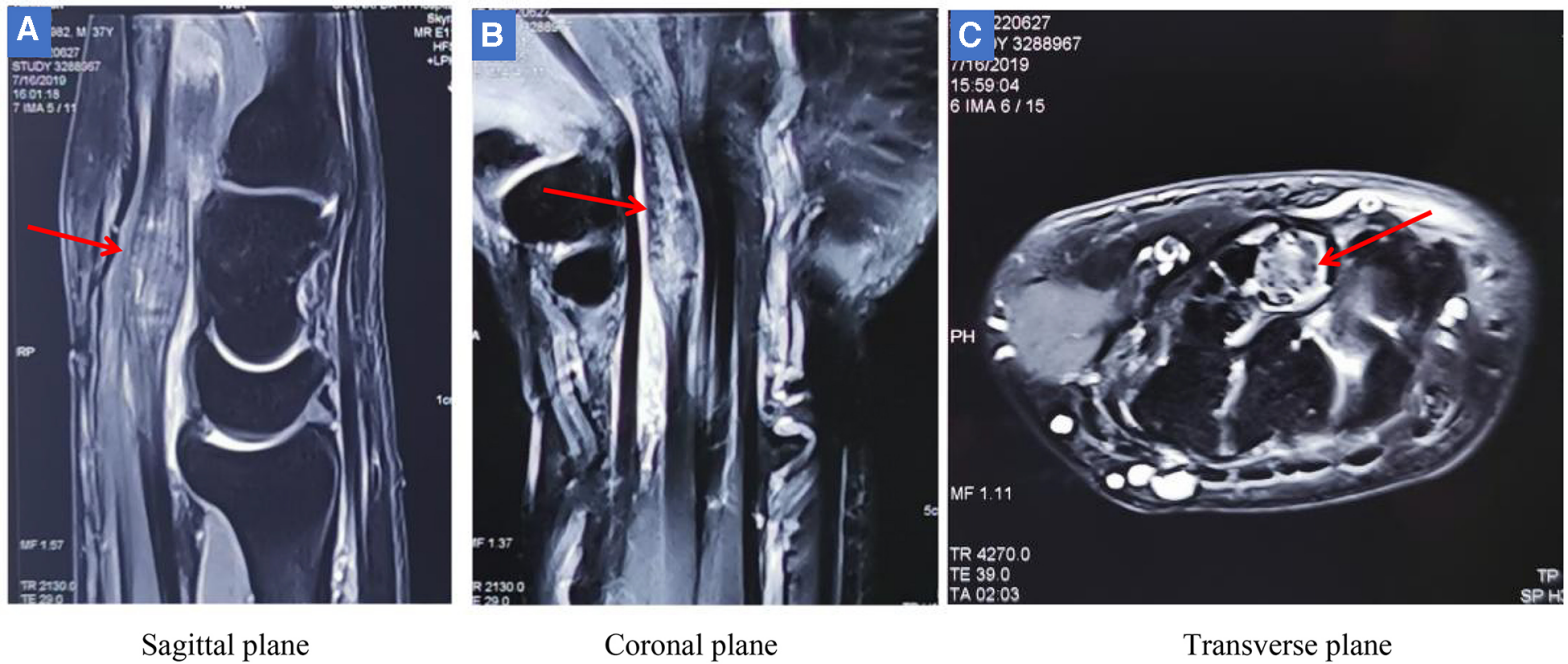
MRI showed that there was a fusiform mass on the palm side of the carpal bone (red arrow). (A-B) The mass was located under the transverse carpal ligament and in front of the carpal bone, and was in the shape of a pike, measuring approximately 0.66 cm 1.37 cm. (C) The mass can be seen in cross section compressing the median nerve. Given the patient's high uric acid levels and MRI findings, it was hypothesized that the mass could be tophi causing carpal tunnel syndrome.
